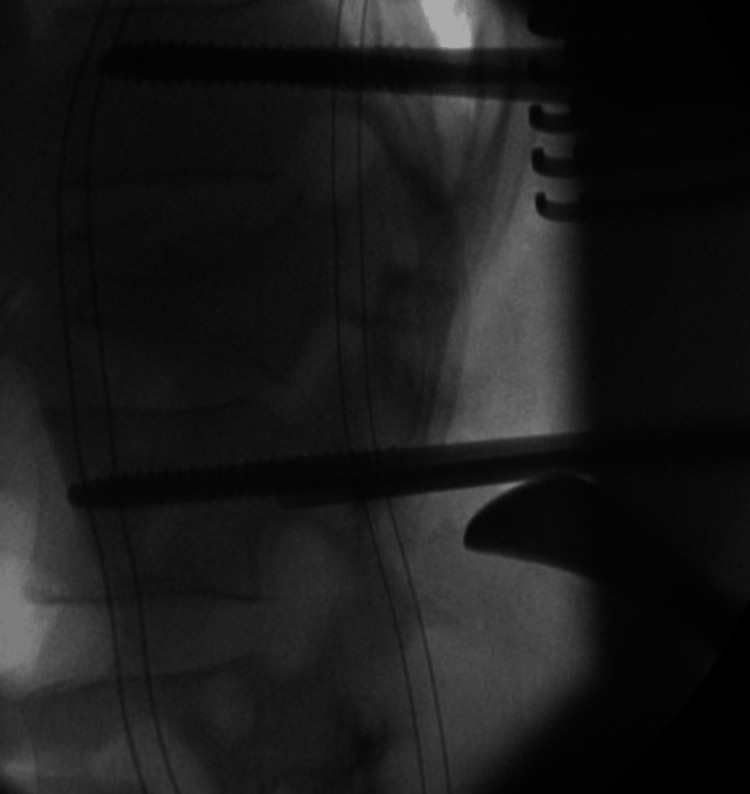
Intraoperative X-rays of open Schanz screw fixation
